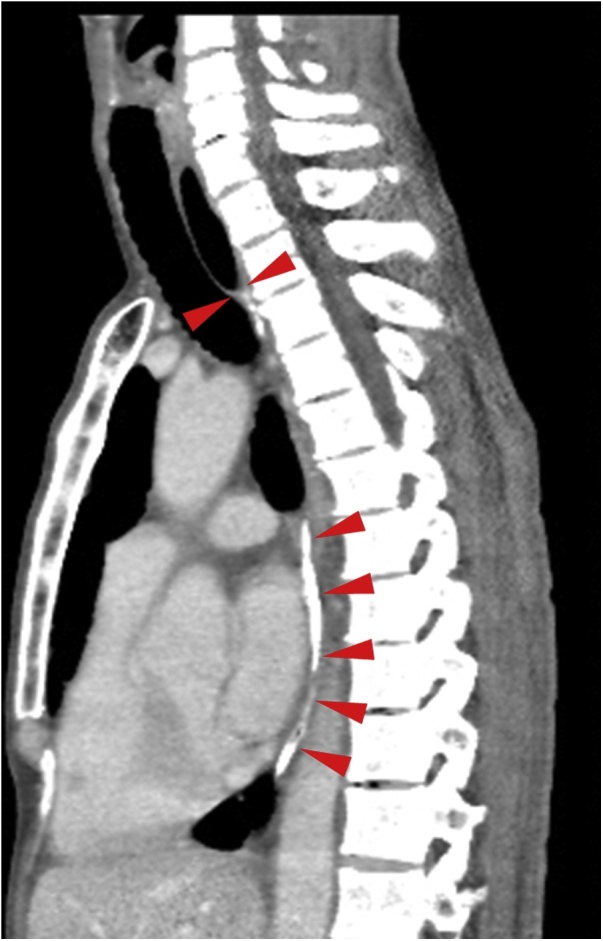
Thoracoabdominal CT (sagittal plane) showing a blind end formed by the cervical esophagus at the superior margin of the sternum and apparent scarring, stenosis, and calcification of the lumen of the thoracic esophagus (arrowhead: stenotic esophagus).
